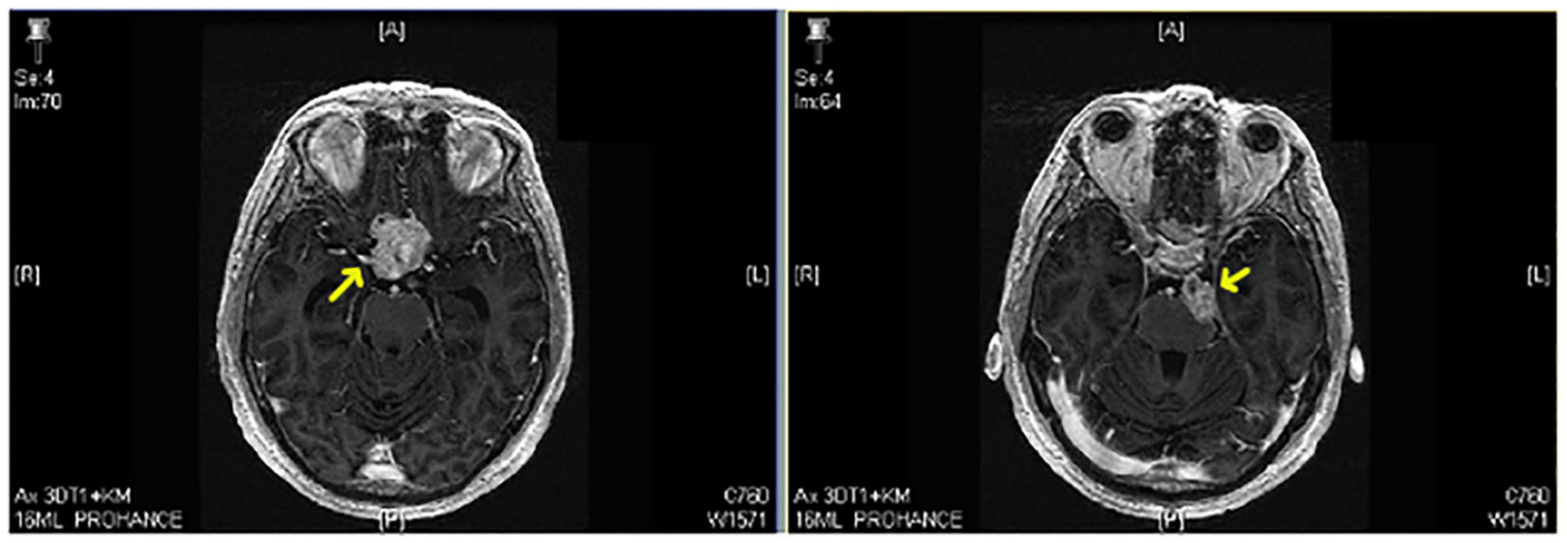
Contrast-enhanced MRI showing an optic nerve hemangioblastoma (yellow arrow) (patient number 12).
